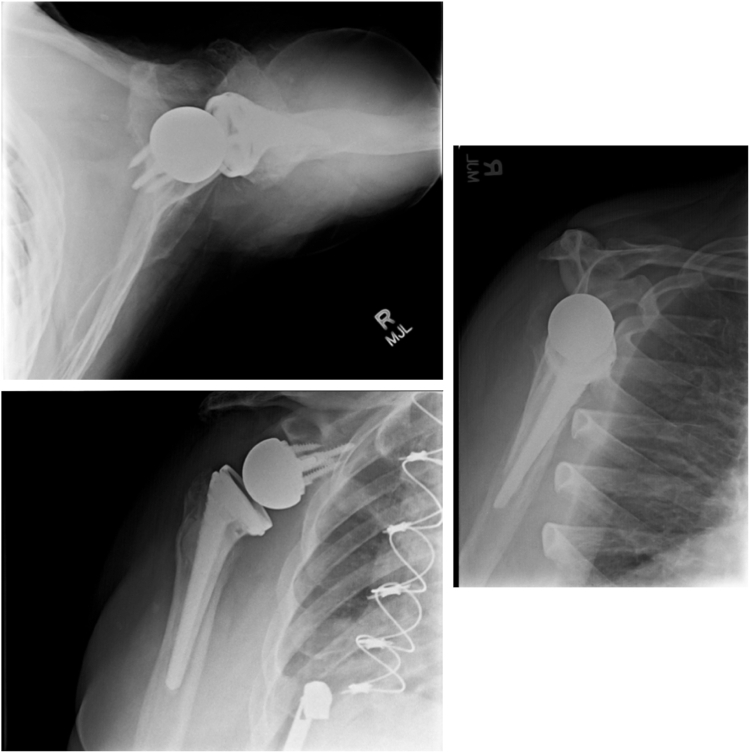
Radiographs of the final reverse total shoulder arthroplasty.
